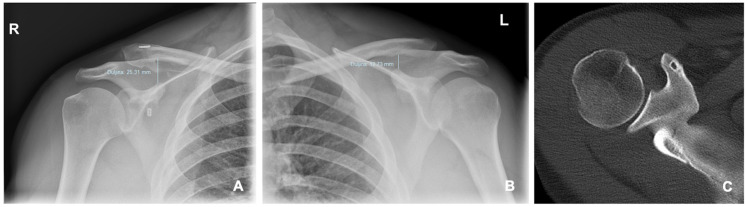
Radiographic and CT assessment of the AC joint. (A,B) Zanca radiographs showing measurement of the CCD between the superior cortex of the coracoid and the inferior cortex of the clavicle on the operated (right) and contralateral (left) side. The CCD is increased on the operated side (25.31 mm vs. 12.73 mm). Measurements were obtained using a Croatian language ISSA 4.0 PACS software (VamsTec d.o.o., Zagreb, Croatia); “Duljina” denotes length (system language setting). (C) Postoperative axial CT demonstrating anterior placement of the coracoid tunnel, distant from the coracoid base, with limited surrounding bone stock.
